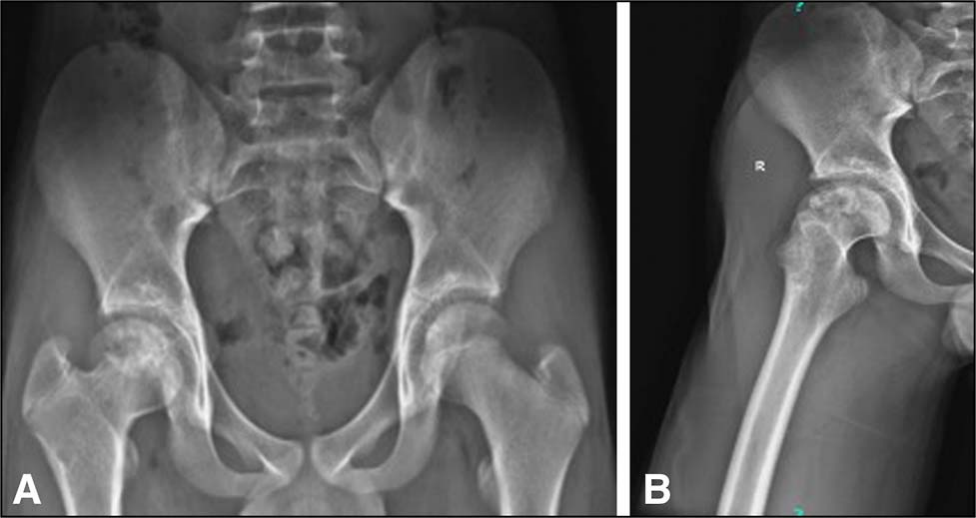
AP radiographs of the (A) pelvis and (B) lateral right hip 5 years after surgery show a concentric femoral head without evidence of recurrence and early closure of the epiphysis.
